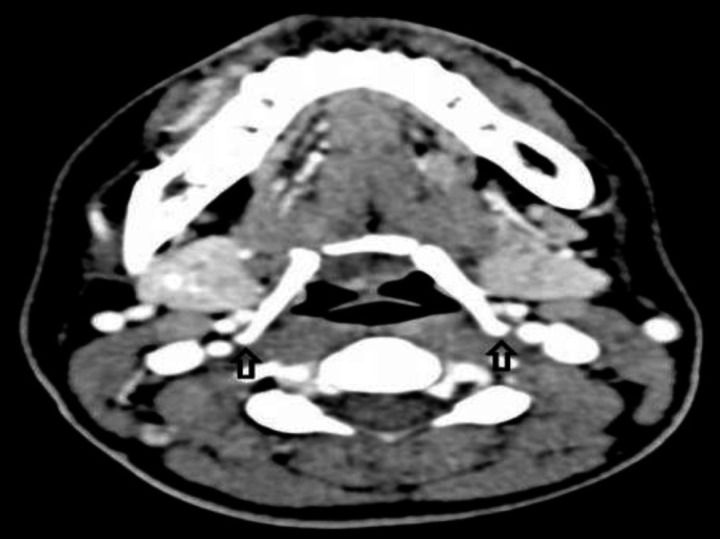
Computed tomography revealed bulky greater horns of the hyoid bone which is in close proximity with body of fourth cervical vertebrae
